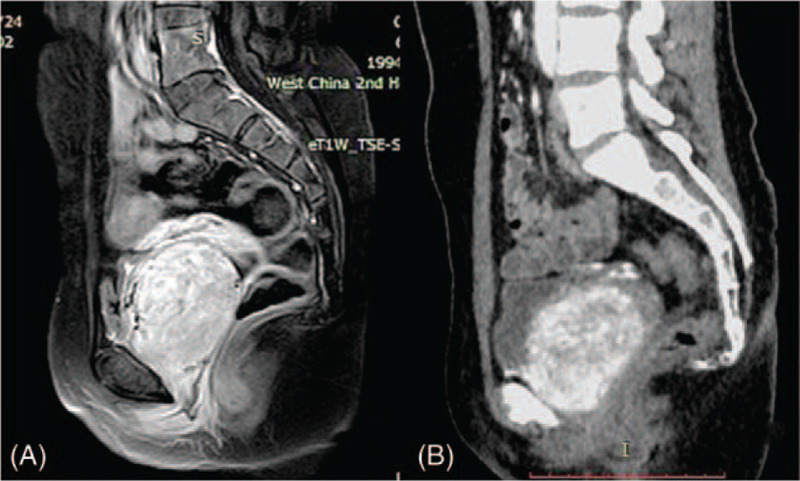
MRI (A) and CT (B) showed a mass on the anterior vaginal wall.
